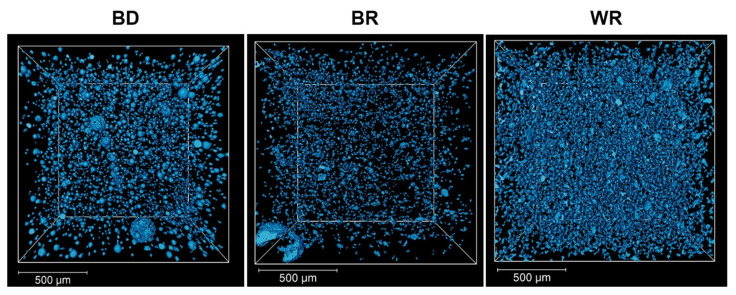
Volume rendering of the segmented pores (blue color) in Biodentine™ (BD), MTA Biorep (BR), and Well-Root PT (WR) obtained by X-ray tomography analysis. The scale bar corresponds to 500 µm in all the images.
